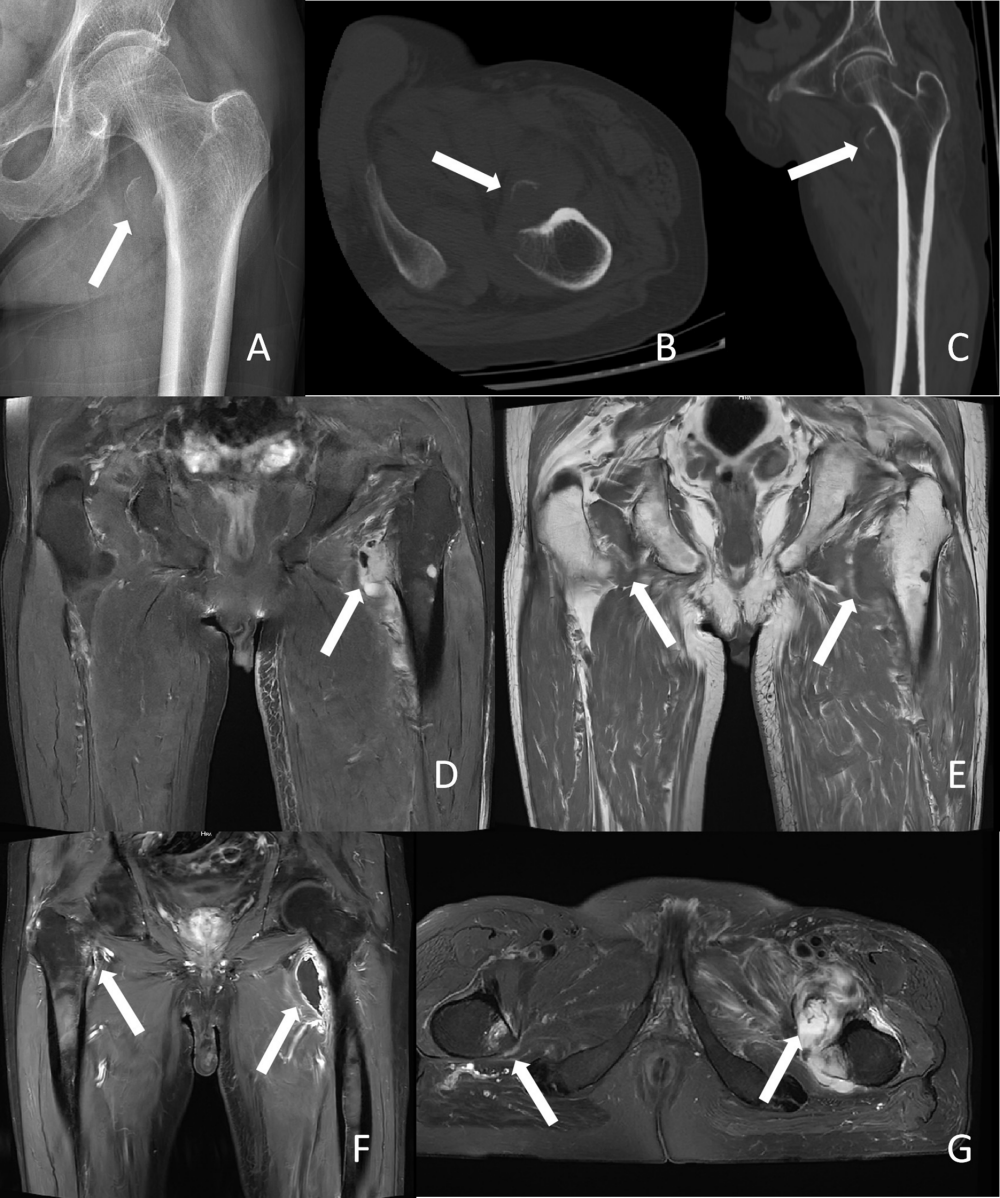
Radiological images of the patient. (A) X-ray findings. The white arrow indicates the fracture site of the left lesser trochanter. (B, C) Computed tomography scan results. The white arrow indicates the fracture site of the left lesser trochanter. (D) Magnetic resonance imaging (MRI) T2-weighted imaging results. The white arrow indicates destruction of the left lesser trochanter, suggestive of a pathological fracture. (E) MRI T1-weighted imaging results. The white arrow indicates both lesser trochanters, suggestive of metastatic tumors in both lesser trochanters. (F, G) Contrast-enhanced MRI results. The white arrow indicates both lesser trochanters, suggestive of metastatic tumors in both lesser trochanters.
